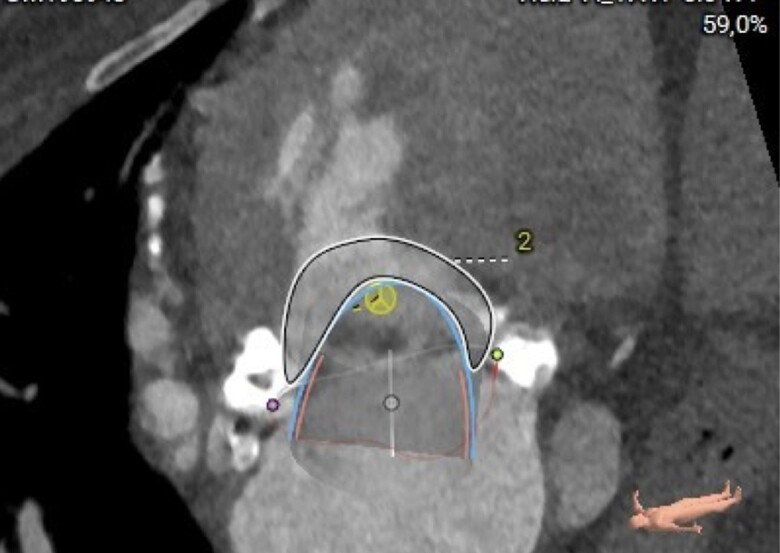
Procedural planning with computed tomography scan and assessment of neo-left ventricle outflow tract of 329 mm^2^ (indicated by the area with number 2).
